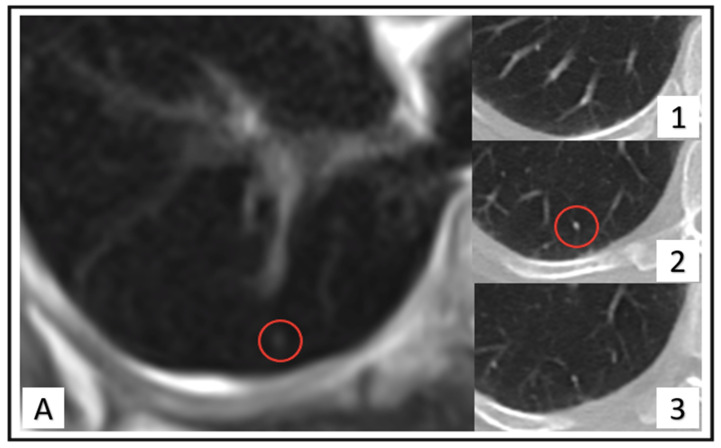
Images of a 62-year-old woman. Three-millimeter lung nodule located in right lower quadrant (red circle) identified at HASTE sequence (A) that turned out as vessel in CT (1–3), continuous structure) due to slice-thickness artifact, evaluated as false-finding.
